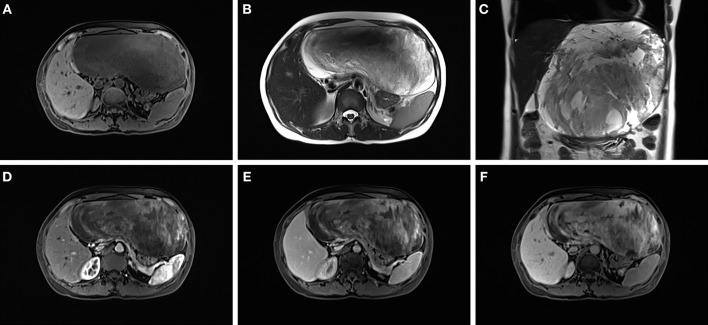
The lesion showed a slightly decreased signal intensity on T1-weighted images (A) and a slightly increased signal intensity on T2-weighted images (B,C). A progressive uneven enhancement was observed after the infusion of contrast material (D–F).
